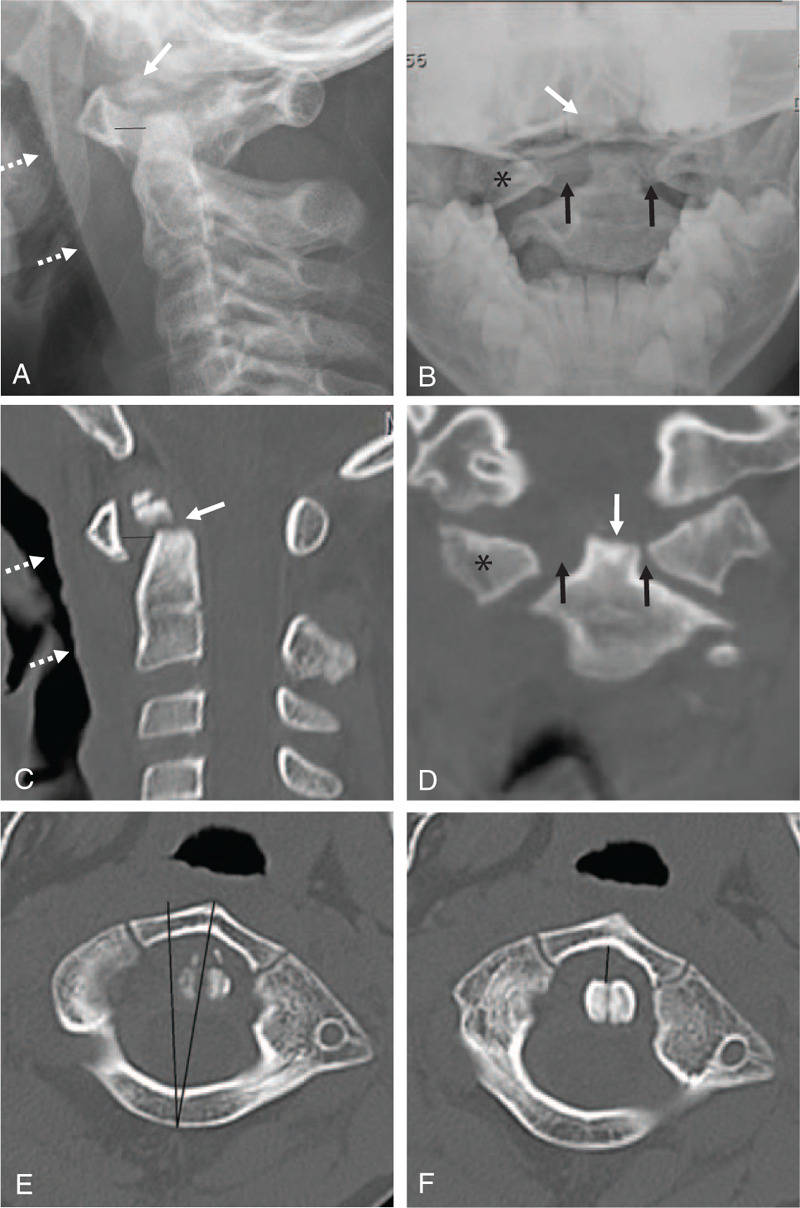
Lateral radiograph (A) showing anteriorly displaced transverse dens fracture along with ossiculum terminale (white arrow), increased atlanto-dental interval (ADI) of 4.5 mm (dark line), and increased anterior soft tissue swelling (dotted white arrows). Open mouth view (B) showing transverse dens fracture (white arrow), asymmetric distance between the lateral mass and dens (dark arrows), and anteriorly displaced right lateral mass of C1 (asterisk). Sagittal (C) and coronal (D) reconstructed computed tomography (CT) scans showing the same finding s more clearly. Axial CT (E and F) scans clearly showing anteriorly displaced and rotated right lateral mass of C1 to the left side and increased ADI of 4.5 mm, which indicating type II atlanto-axial rotatory subluxation (AARS) (according to Fielding and Hawkins classification).
